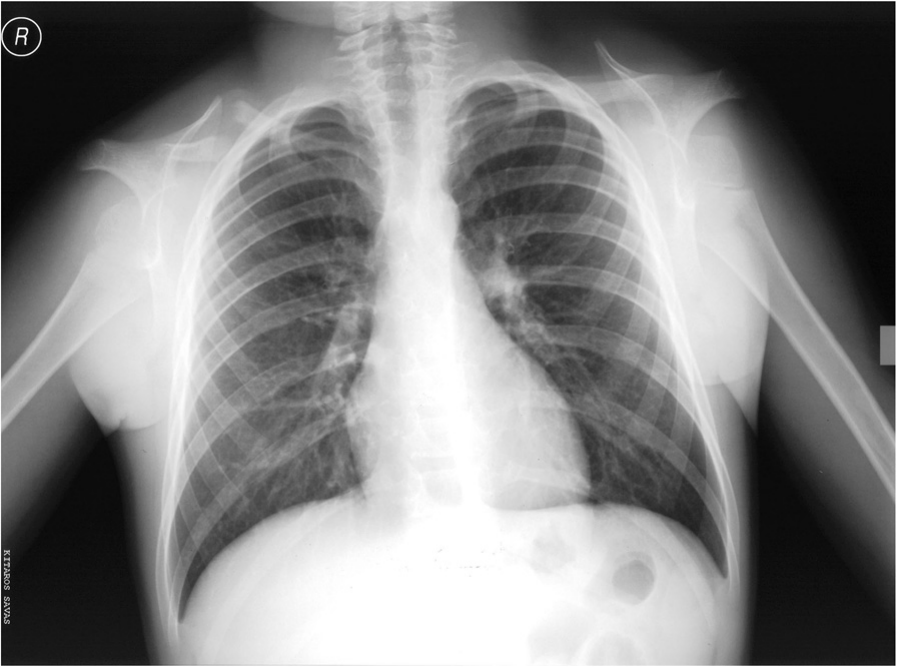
Chest radiograph showing hypoplastic clavicles. (From Kolokitha OE, Papadopoulou AK [1] with permission).
